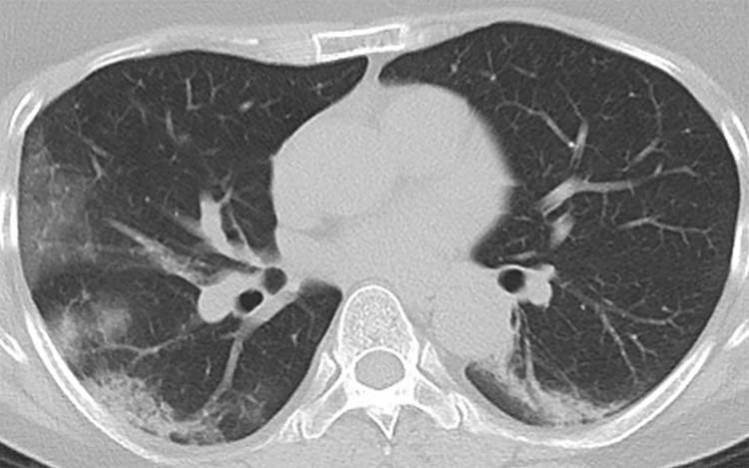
Chest CT scans showed multifocal bilateral peripheral ground-glass opacities.
